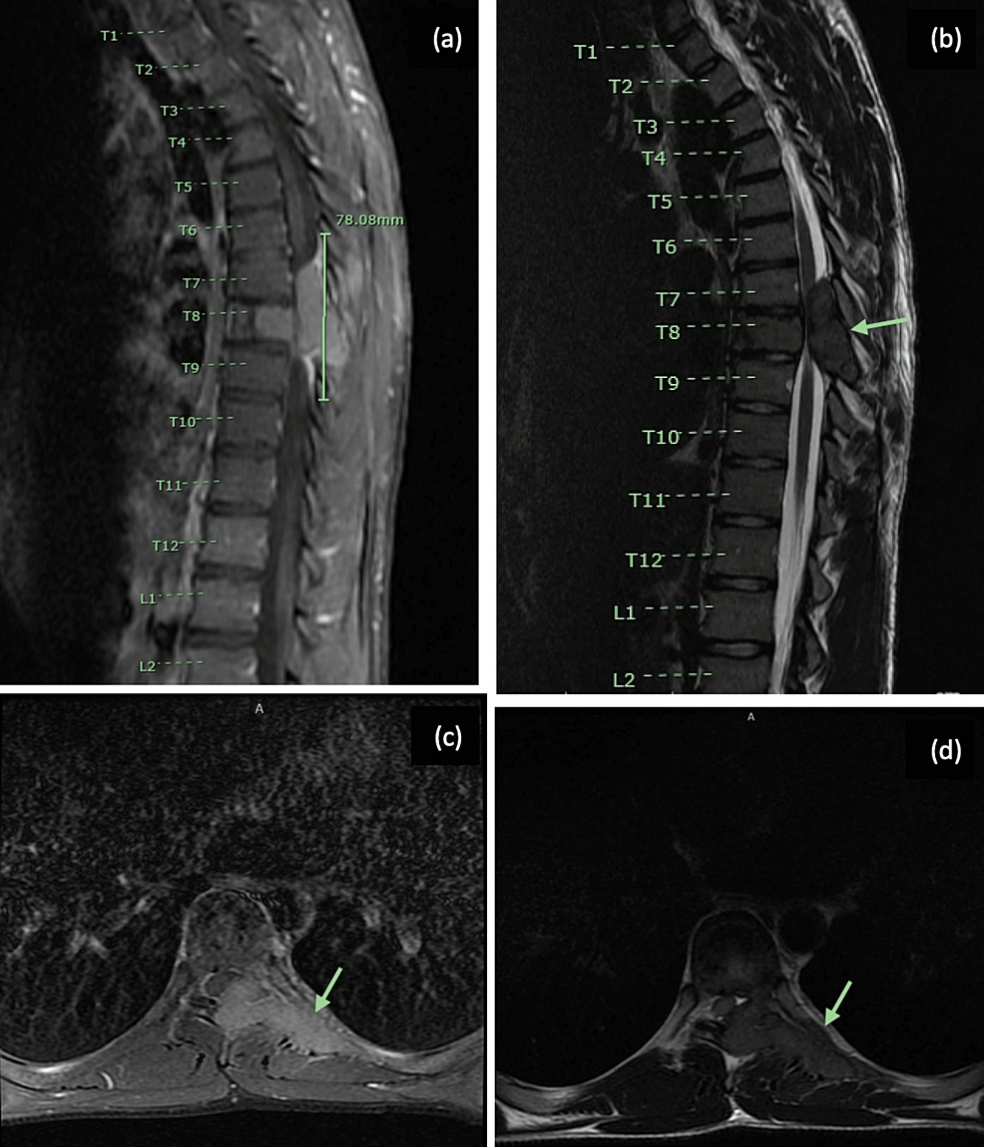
Pre-operative MRI showing a diffuse, marrow-replacing lesion at T8 with resulting effacement into the T7-T8 CSF spaces and severe spinal cord compression. (a) Sagittal T1-weighted fat-saturated post-contrast image. (b) Sagittal T2-weighted image. (c) Axial T1-weighted fat-saturated post-contrast image. (d) Axial T2-weighted image.
